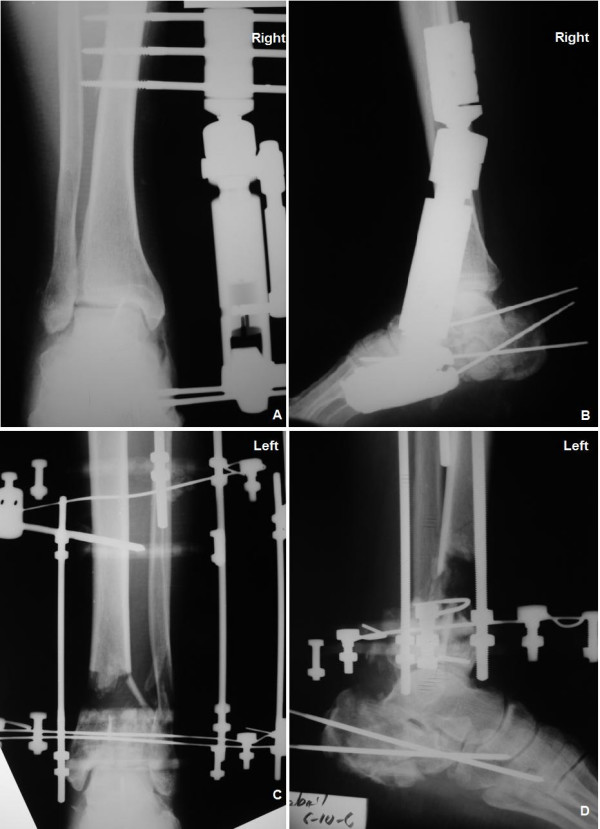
Early postoperative anteroposterior and lateral radiographs of both feet.
